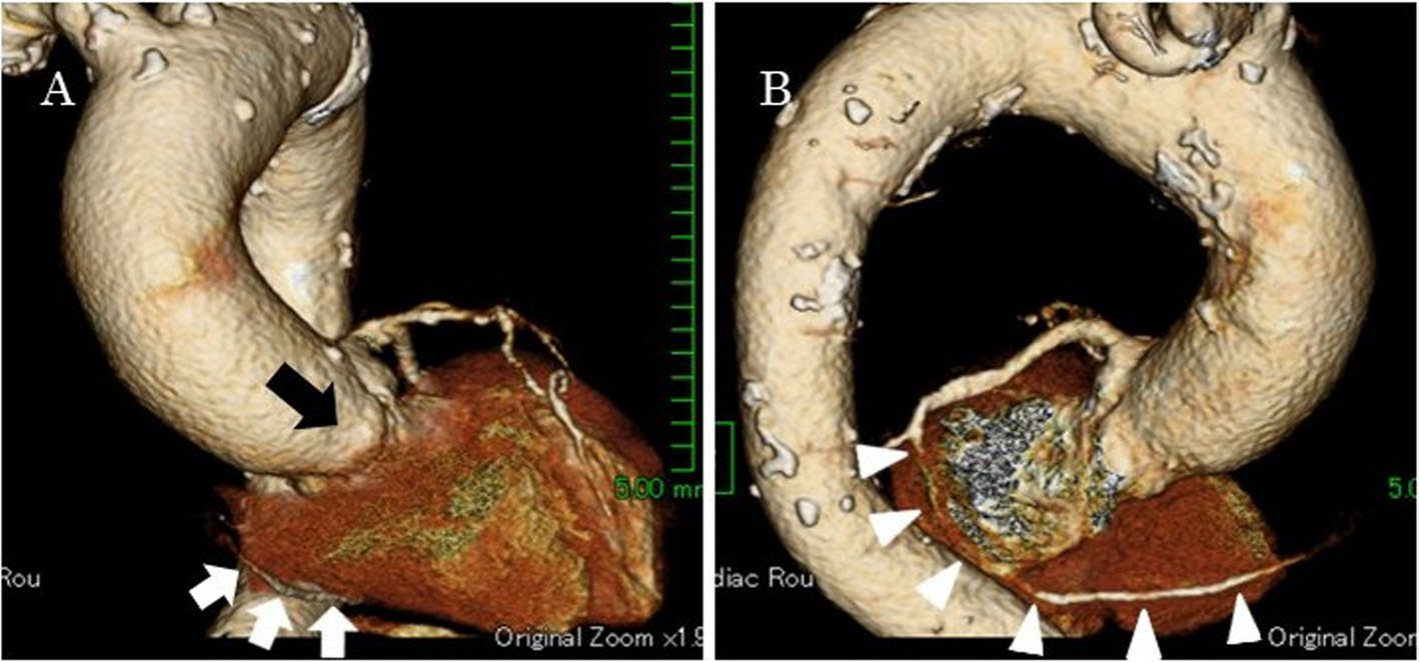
3D computed tomography image taken during conservative therapy. A Front view. The white arrows indicate the coronary artery continuing from distal segment of the left circumflex artery. The right coronary artery is absent at the Valsalva sinus, as indicated by the black arrow. B The arrowheads show the distal segment of the left circumflex artery continuing to the right coronary artery at the atrioventricular groove
